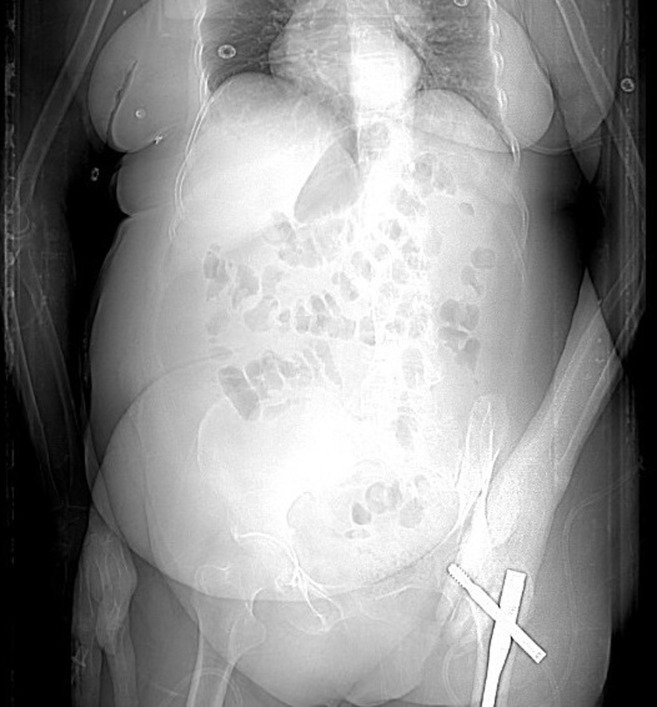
postoperative radiograph showing internal fixation of a pertrochanteric femoral fracture
